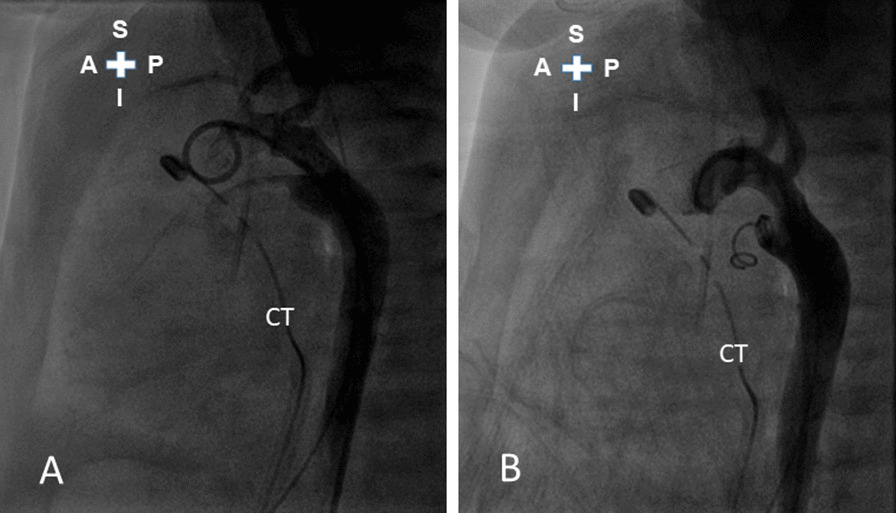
a Lateral-view aortogram showed a Krichenko Type A1 patent ductus arteriosus with the narrowest portion 1.7 mm in diameter; b Lateral-view aortogram after the implantation of a Gianturco coil (MWCE-38-6-4) showed complete closure of the patent ductus arteriosus without residual shunting, but approximately half of the coil was extruded into the pulmonary artery with a resultant redundant and bulky appearance. *A* anterior; *CT* chest tube; *I* inferior; *P* posterior; *S* superior
